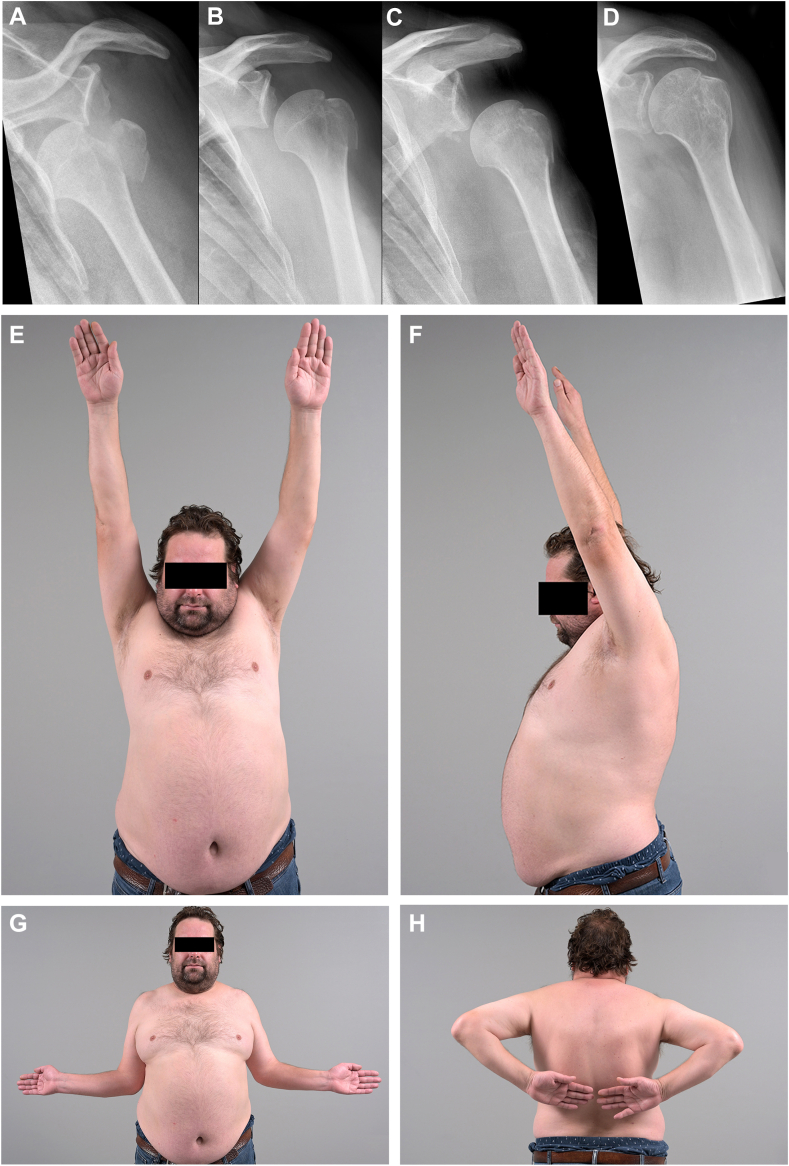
(A) ap x ray showing ASD with avulsion fracture of GT. (B) ap x ray after closed reduction with reduced GT fragment and HHPS. SSV 0%. (C) 6 weeks after ASD and closed reduction with severe HHPS and healed GT fragment. SSV:15%. (D) ap x-ray 10 months after ASD and closed reduction showing recentered humeral head and normalized impingment indices. SSV: 99%. (E-H) photographic documentation of ROM at last follow-up (10 months). Flexion 160° (E and F), external rotation 70° (G). internal rotation L3 (H). *ASD*, anterior shoulder dislocation; *GT*, greater tuberosity; *SSV*, subjective shoulder value; *HHPS*, humeral head pseudosubluxation; *ROM*, range of motion.
